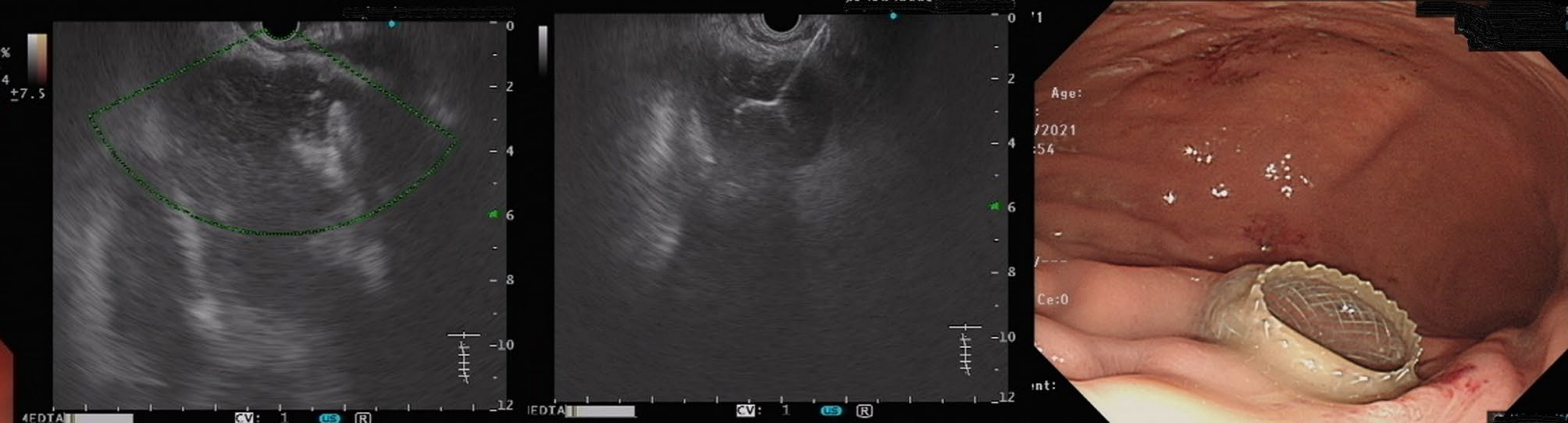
Endoscopic ultrasound (EUS)-guided deployment of a lumen-apposing metal stent (left & middle: EUS imaging; right: endoscopic imaging).
